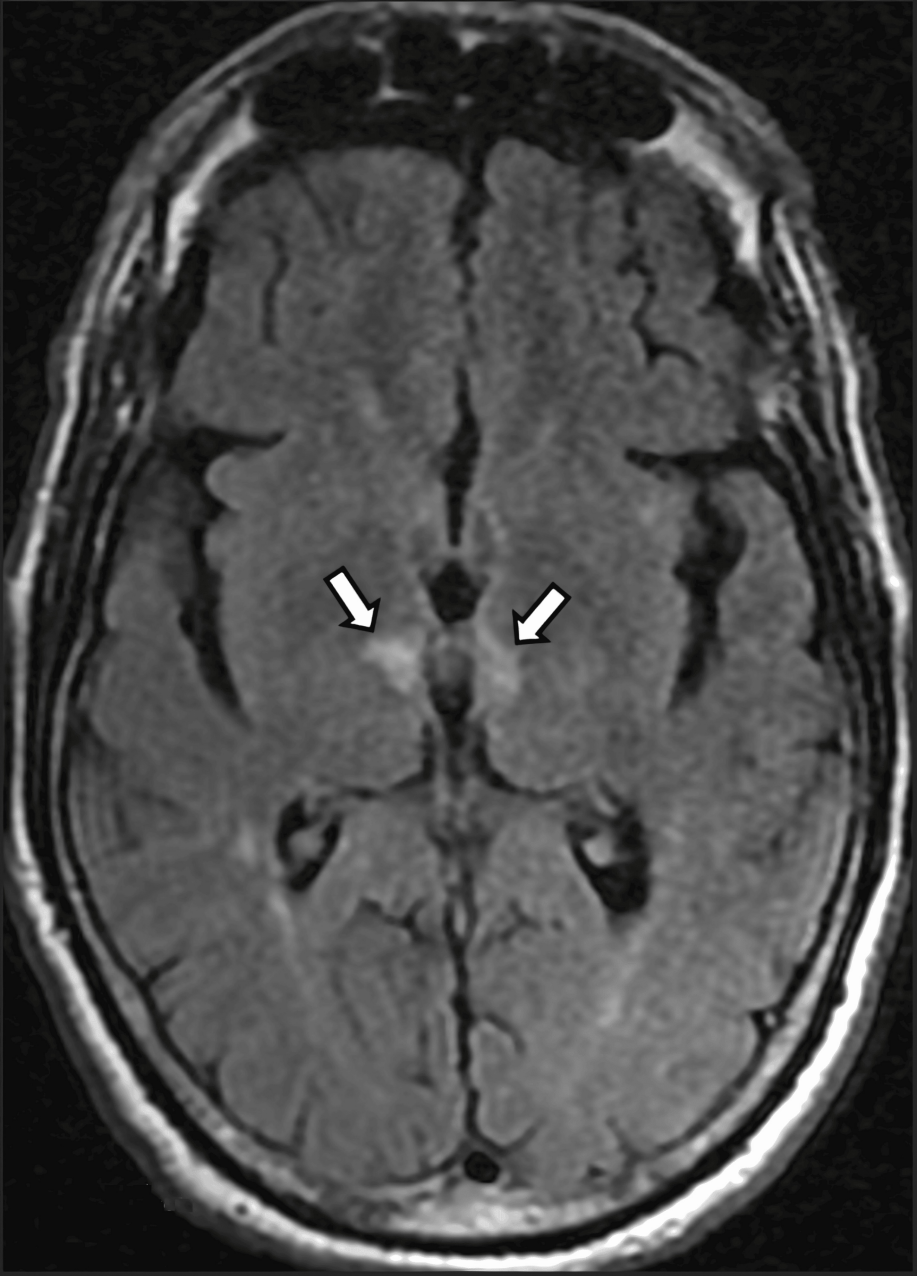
Bilateral paramedian thalamic hyperintensities in FLAIR sequences (white arrows) were consistent with the diagnosis of AOP infarction. AOP: artery of Percheron; FLAIR: fluid-attenuated inversion recovery
